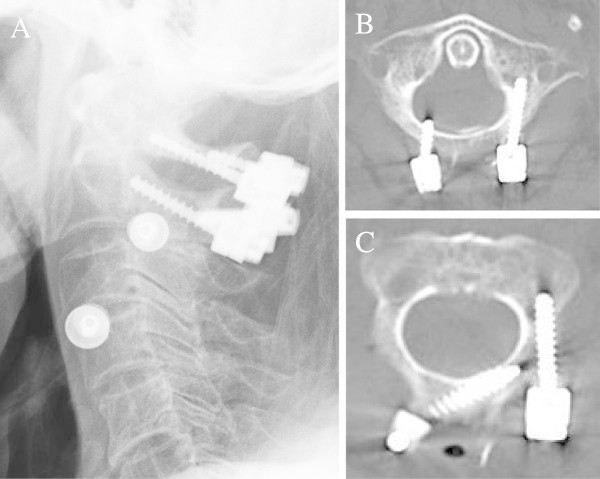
X-ray and computed tomography (CT) images after surgery. Radiography revealed posterior fixation and reduced C1-C2 dislocation (A). Axial CT images showed posterior arch screw and lateral mass screw at C1 (B), and intralaminar screw and pars interarticularis screw at C2 (C).
